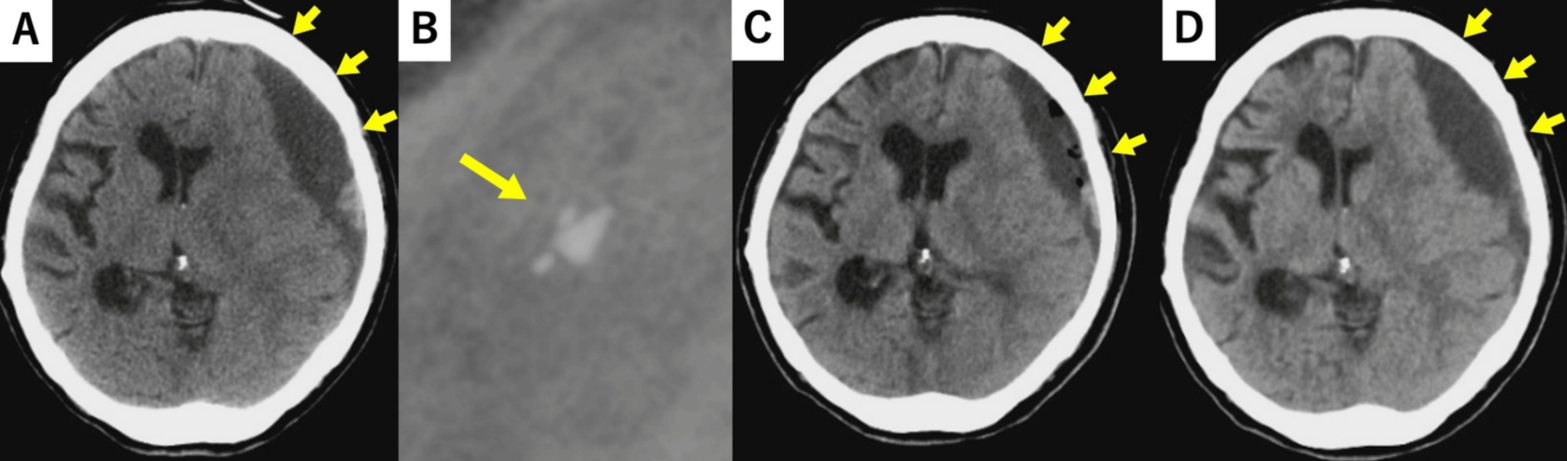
A: Plain CT axial image of the head on admission; B: Plain radiograph of the head on admission; C: CT immediately after burr hole hematoma removal; D: CT on the fourth day after the initial surgery A: Left chronic subdural hematoma with midline shift was observed. B: The shape of the valve marker revealed it as a fixed valve (NovusTM Valve, Natas, USA). C: Temporary decrease in hematoma observed. D: The hematoma had re-enlarged, and the midline shift had worsened.
